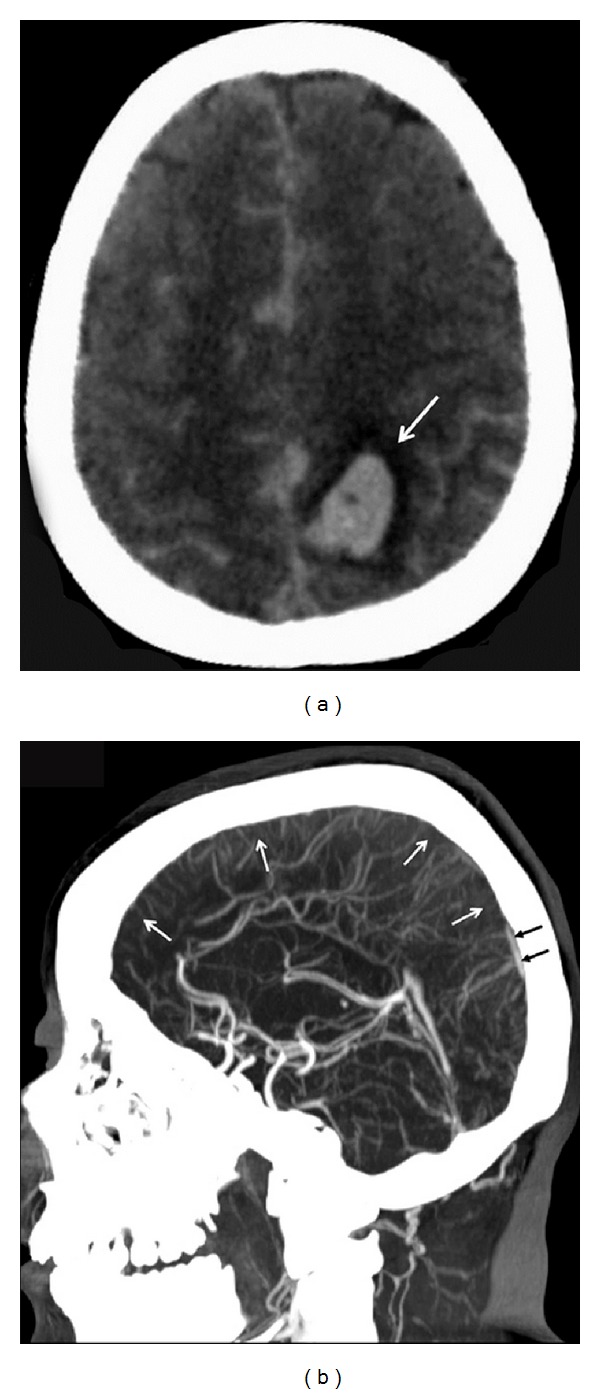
(a) Hemorrhagic area (arrow) is observed on the edematous background at bilateral frontal and parietal regions on noncontrast CT. (b) On sagittal reformat CT angiograms, a thrombus completely filling superior sagittal sinus (white arrows) is observed. Partially confluent sinus (black arrows) and transverse sinus surface patterns are seen.
